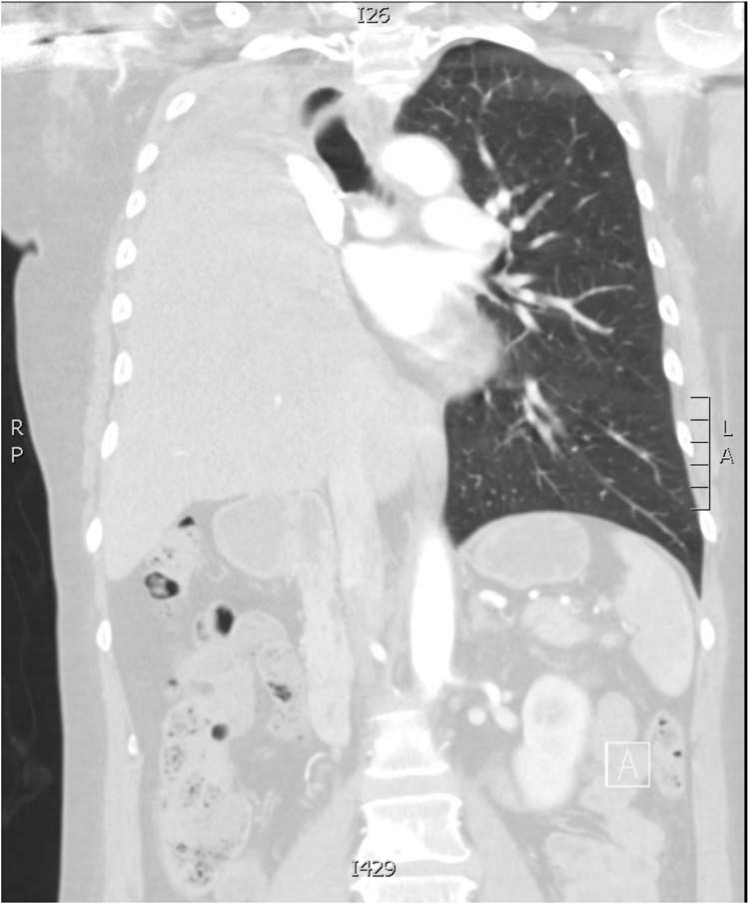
Follow-up CT imaging demonstrating complete migration of the liver into the right thoracic cavity.
